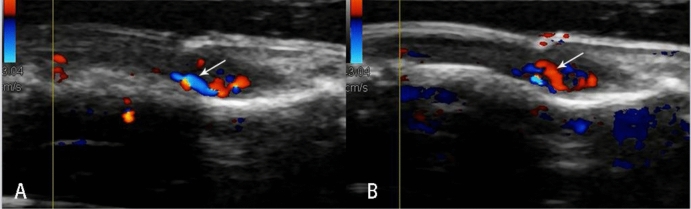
Color Doppler imaging shows abundant blood flow signals in the Subungual glomus. Arrows note the afferent artery (A, blue one) and the efferent vein (B, red one).
